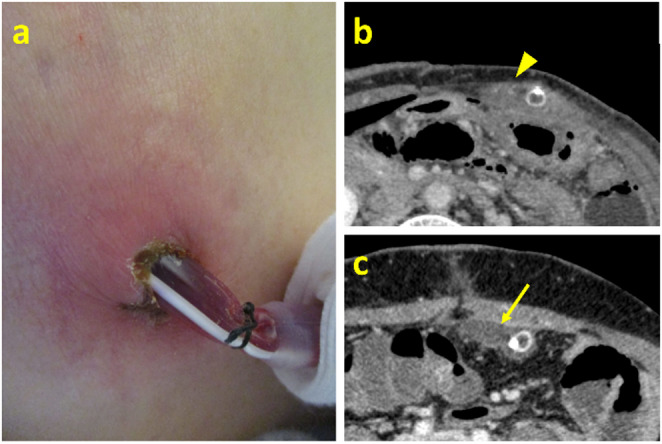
Representative findings of retrograde drain infection. (a) Photograph of the drain insertion site showing redness and swelling. (b) Computed tomography (CT) scan image showing inflammation of the soft tissue and muscle around the drain, classified as deep surgical site infection (SSI) (arrowhead). (c) CT scan image showing intraabdominal inflammation and fluid collection along the drain insertion route, classified as organ/space SSI (arrow)
